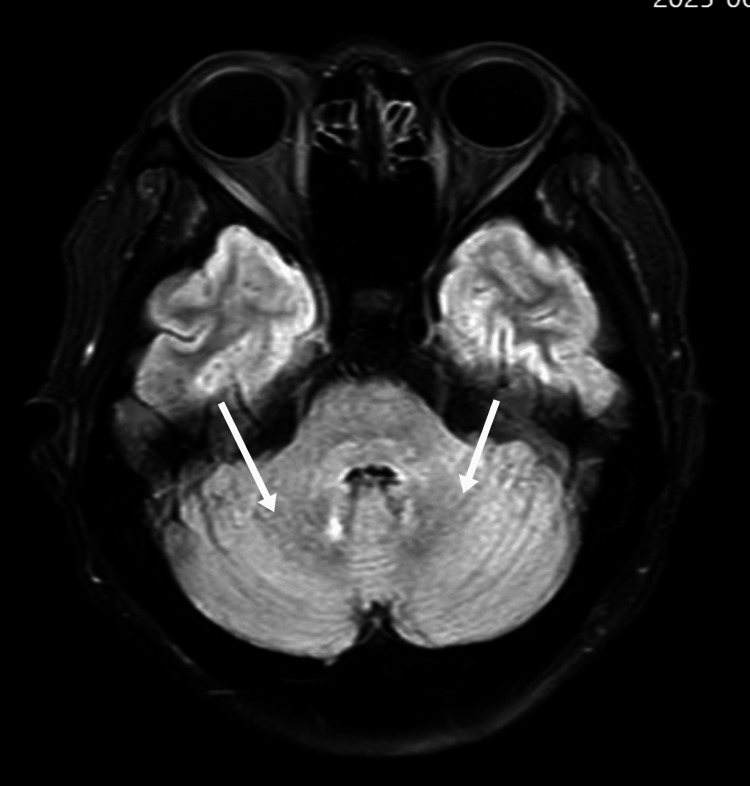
Axial FLAIR MRI of the cerebellum after treatment As indicated by the white arrow, the follow-up axial T2-FLAIR image demonstrates near-complete resolution of the previously observed bilateral hyperintense lesions in the cerebellar dentate nuclei FLAIR: fluid-attenuated inversion recovery; MRI: magnetic resonance imaging
